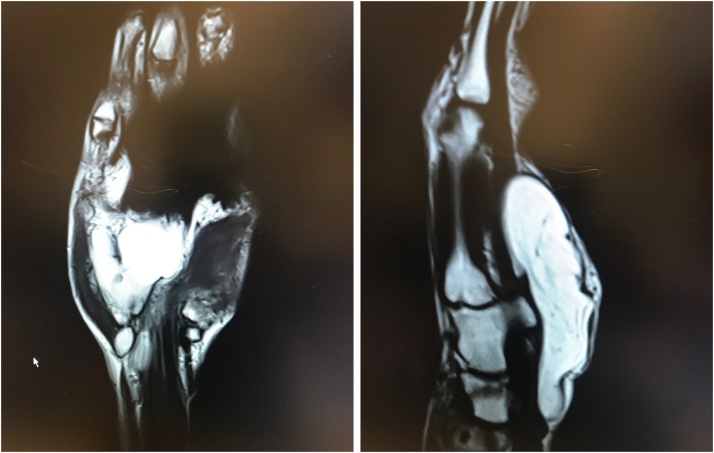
MRI-scan of the hand depicting the fatty tissue tumour. The mass was localized ventrally from the flexor tendons, measuring 4,8 × 1,8 cm in the transverse plane. In the sagittal plane, the maximum diameter was 8,6 cm.
